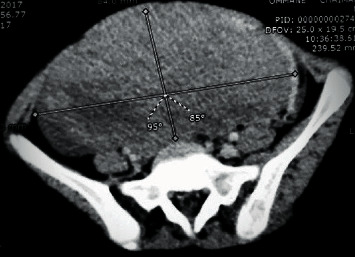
Contrast-enhanced CT shows a heterogeneous density abdomino-pelvic tumor.
